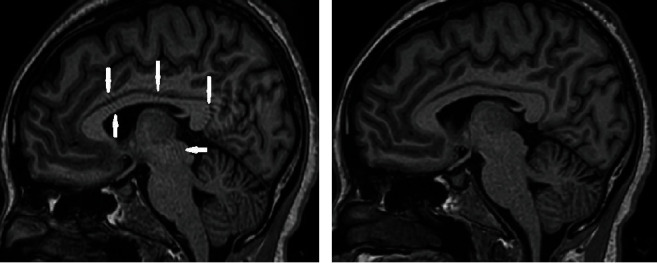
Sagittal 3D T1-weighted image of the brain (a) acquired with sequence parameters equivalent to FreeSurfer protocol: white solid arrows indicating areas of pulse sequence-related artifacts and (b) improved image acquired with the optimized protocol.
